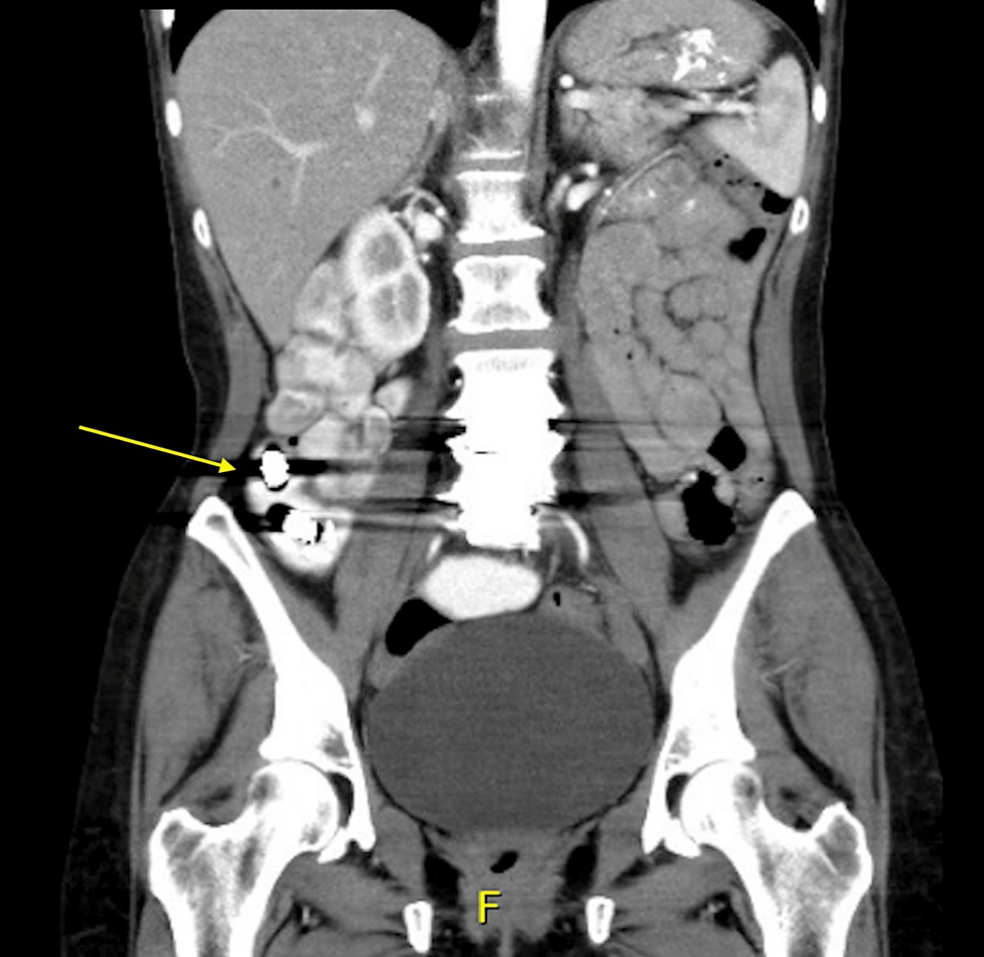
CT coronal view with the arrow pointing to two video capsules in the distal small bowel.
